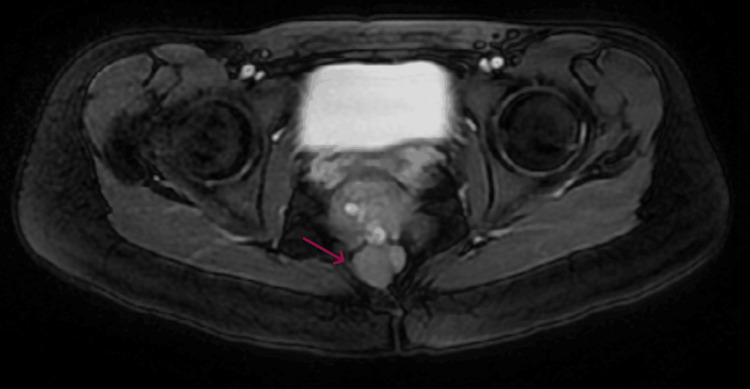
Axial 2D FIESTA MRI demonstrating a mildly enhancing solid lesion anterior to the coccyx, measuring 2.5 × 2 × 1.8 cm (red arrow) 2D FIESTA MRI: two-dimensional fast imaging employing steady-state acquisition magnetic resonance imaging
